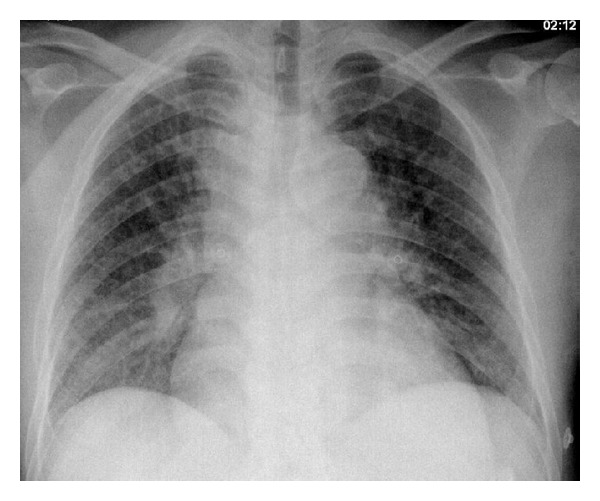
Roentgenogram of chest revealing bilateral reticular infiltrates with mediastinal widening suggestive of enlarged hilar and mediastinal lymph nodes. Enlarged cardiac shadow.
